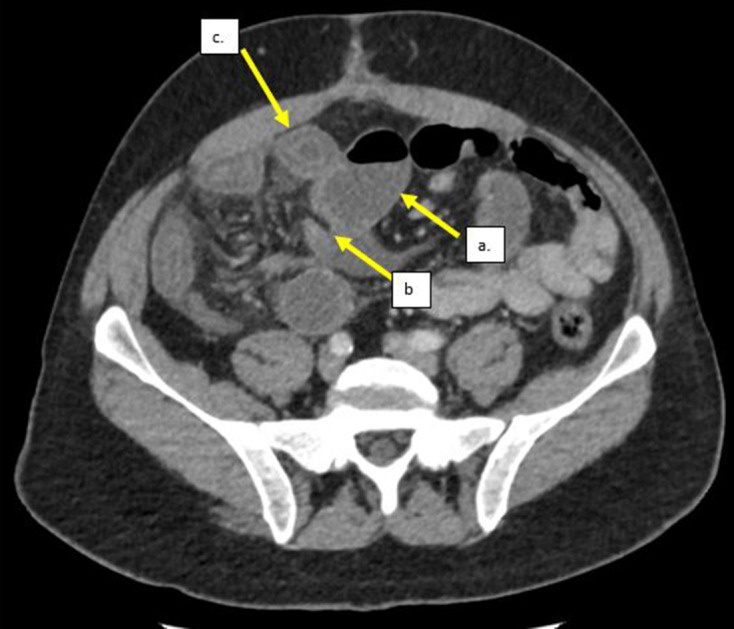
An axial enhanced CT showing: a. Dilated small bowel up stream to the transition point. b. Acute transition point caused by a fat density band indenting the bowel. c. Non-dilated but edematous small bowel downstream to the transition point. This was initially misinterpreted as recurrent Crohns but was re-reported as congestion and edema downstream to the fibrofatty band obstruction.
